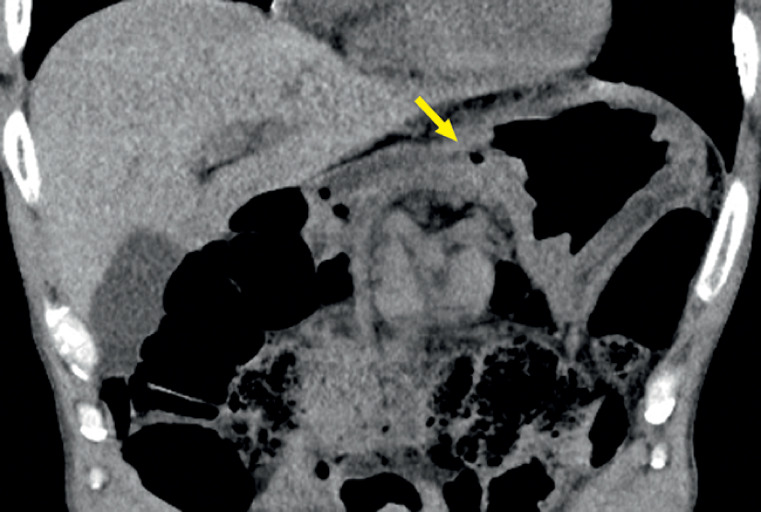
An abdominal computed tomography scan showed the ectopic pylorus opened to the less curvature of stomach (yellow arrow).
